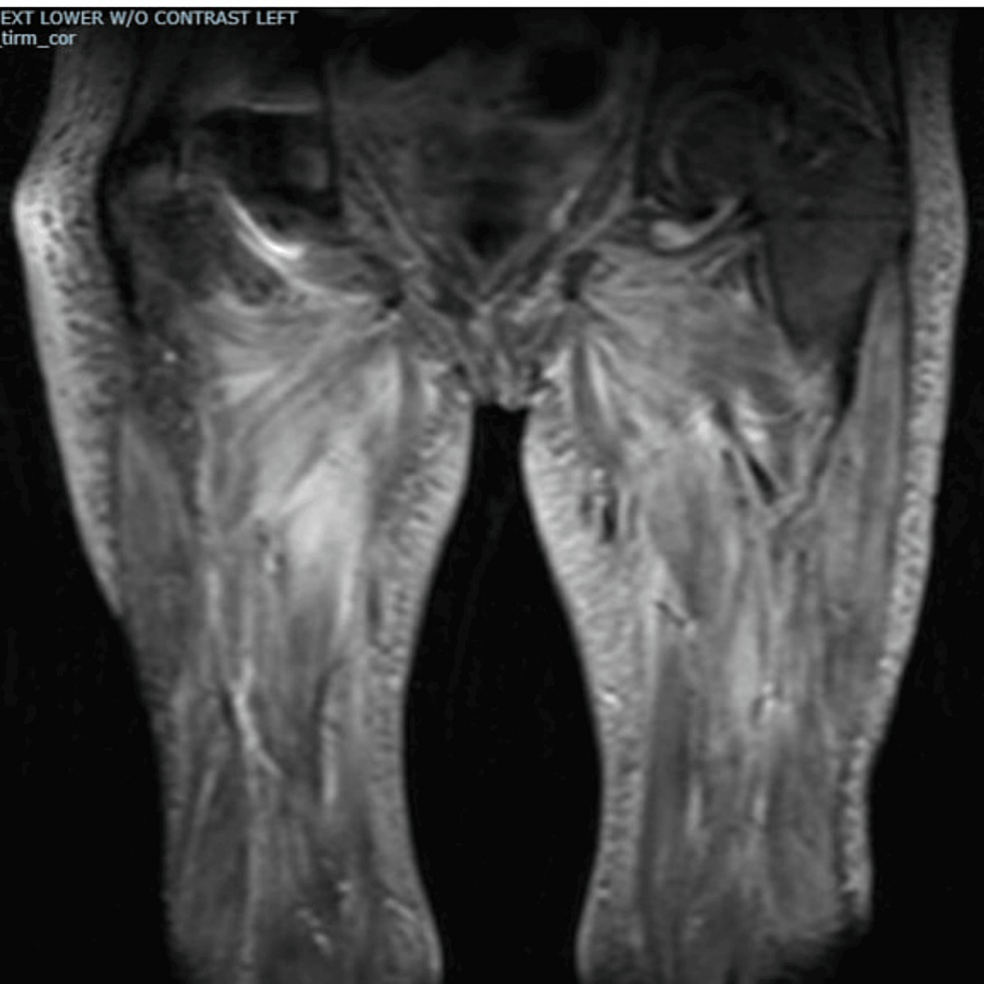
MRI of bilateral thighs showing muscle edema
